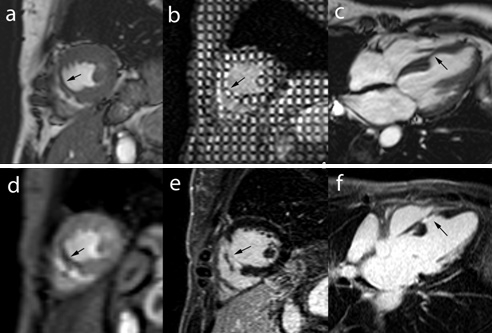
Fig. 9MRI study in a 47 year–old female with LV septal wall motion abnormalities (incidental findings) on echocardiography probably due to an embolic occlusion of a septal LAD coronary artery branch. Midventricular short–axis and horizontal long–axis cine MRI (a,c) and short–axis tagging MRI (b) show marked mid septal wall thinning with dyskinetic wall motion (*arrows*). First–pass perfusion MRI (d) and late ce–MRI (e,f) show a perfusion defect (d) and transmural enhancement (e, f) within the thinned myocardium (*arrows*).
